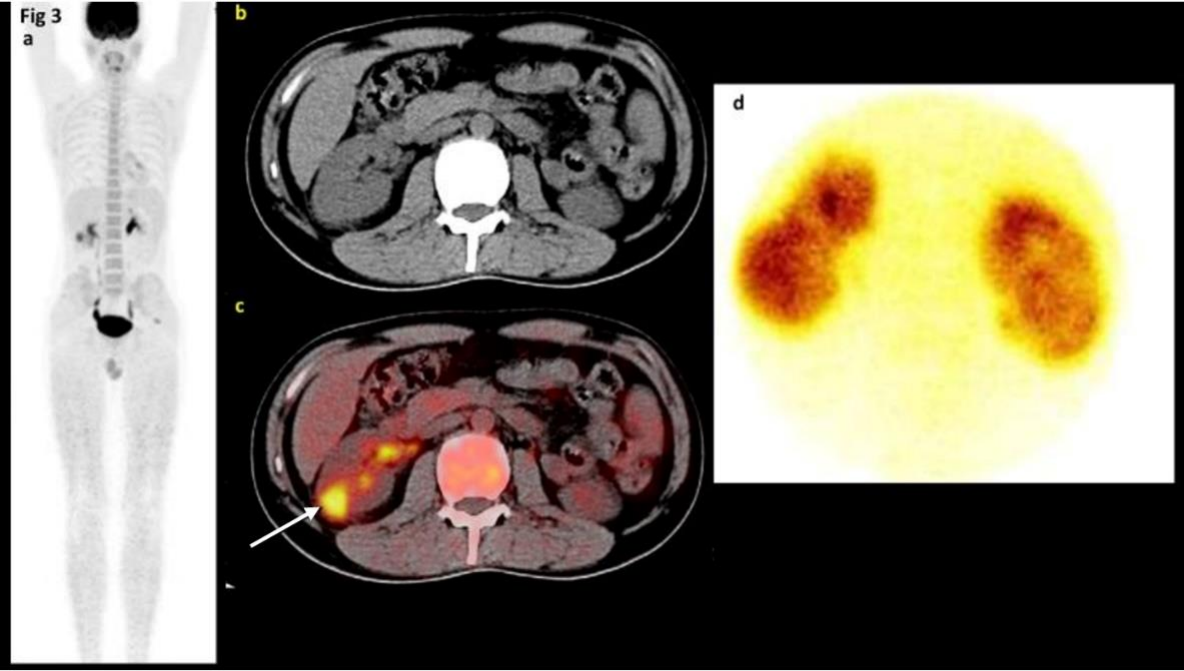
A 38-year-old male with fever and no detectable cause undergoing FDG PET/CT scan (There was a focus of increased FDG uptake in the inferior pole of the right kidney [c]. The ^99m^Tc-DMSA scan showed photon deficiency in the right kidney laterally corresponding to focal FDG uptake compatible with acute pyelonephritis [d]. The left kidney also showed irregular contour suggesting scars.)
